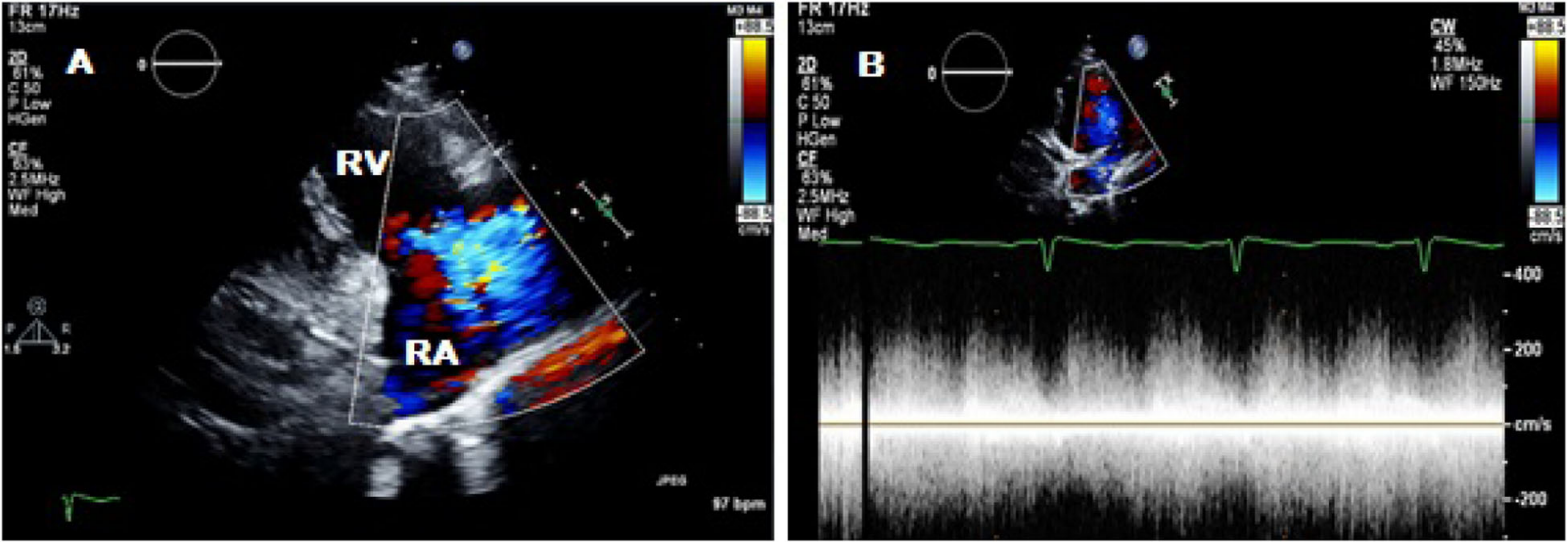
Two-dimensional transthoracic echocardiography in parasternal long axis right ventricular inflow view; a tricuspid valve regurgitation jet on colour Doppler echocardiography. b Continuous wave Doppler through tricuspid valve regurgitation jet showing a continuous flow. RV, right ventricle; RA, right atrium
